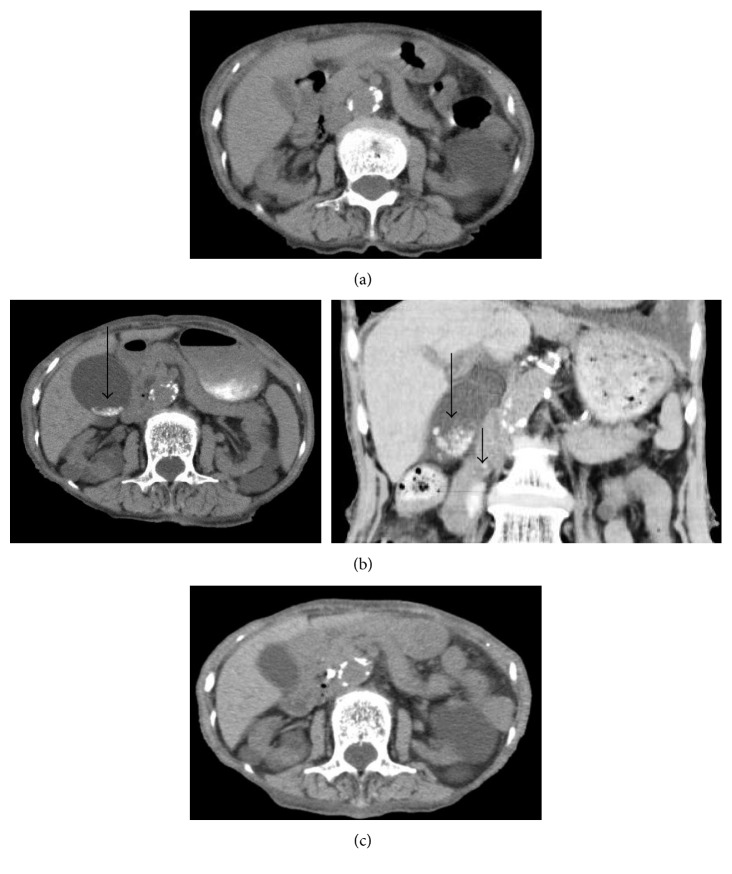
CT scans in Case 1. (a) Small stones or sludge were not detected in the biliary tract before administration of CTRX. (b) (*Left*, axial view; *right*, coronal view) small stones and sludge appeared in the gallbladder (long arrows) and common bile duct (short arrow) 3 days after ending CTRX administration. (c) The small stones and sludge disappeared after 4 weeks.
